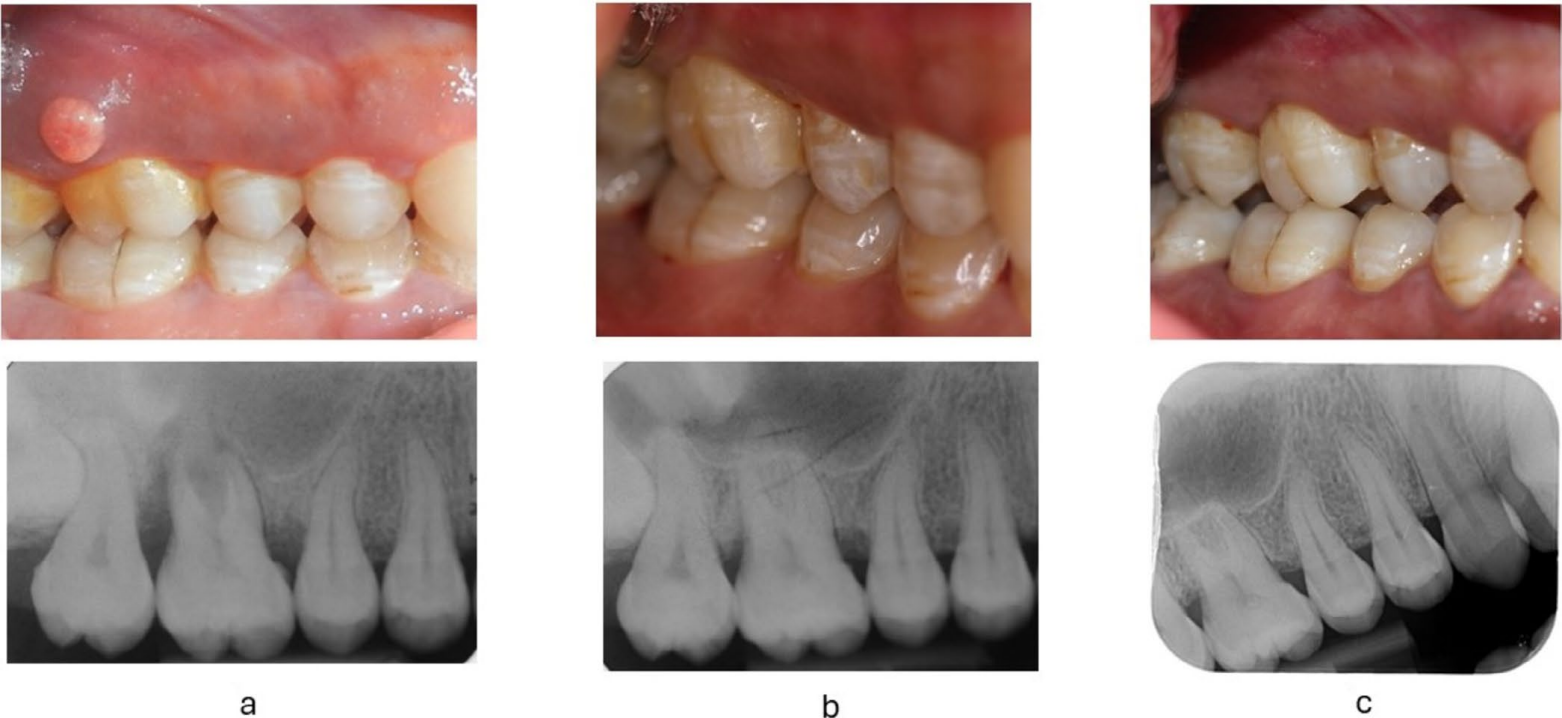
Baseline, 1‐year and 5‐year follow‐up of upper right first molar treated with steps 1 and 2 periodontal therapy, including MINST. The tooth was responsive to sensibility testing at baseline (a) and presented a disto‐buccal PPD of 11 mm coupled with distal furcation involvement degree II. The follow‐ups at 1 year (b) and 5 years (c) show clinical resolution of the pocket (4‐mm pocket depth not bleeding on probing) and furcation (no furcation probing), associated with the closure of the radiographic intrabony defect.
